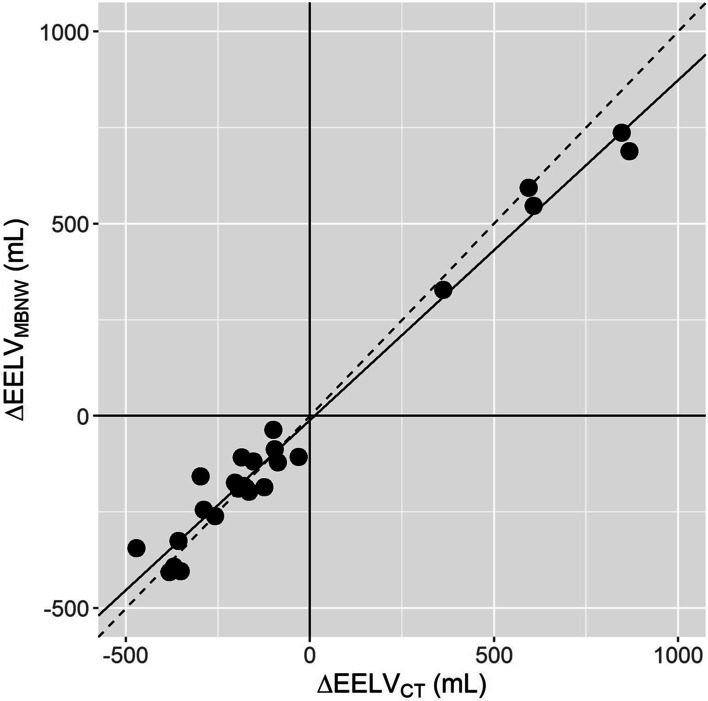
Four quadrants plot relating EELV_MBNW_ and EELV_CT_ changes between consecutive measurements. Horizontal and vertical continuous black lines are quadrant limits. Oblique dashed line is line of identity. Solid line is regression line. Each symbol is the change in end-expiratory lung volume between consecutive measurements assessed with the multiple breath nitrogen washin–washout technique (ΔEELV_MBNW_) and with computed tomography (ΔEELV_CT_)
